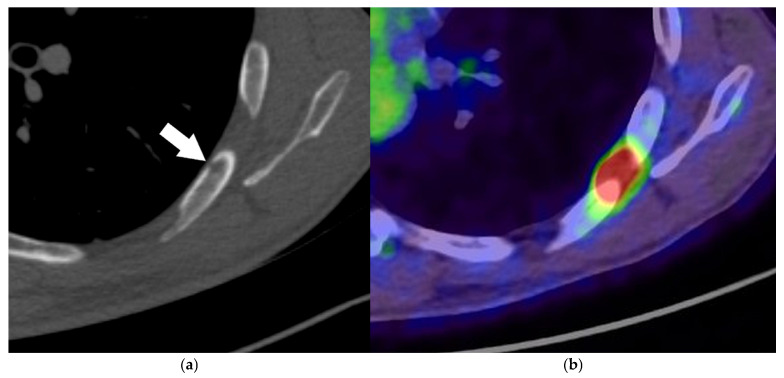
PET/CT bone metastasis detection: Bone window CT scan (width 1800, level 400) showing a subtle rib lytic lesion (arrow) that was not detected during CT analysis (a). High FDG uptake in the bone lesion is evident on PET/CT, confirming bone metastasis (b).
